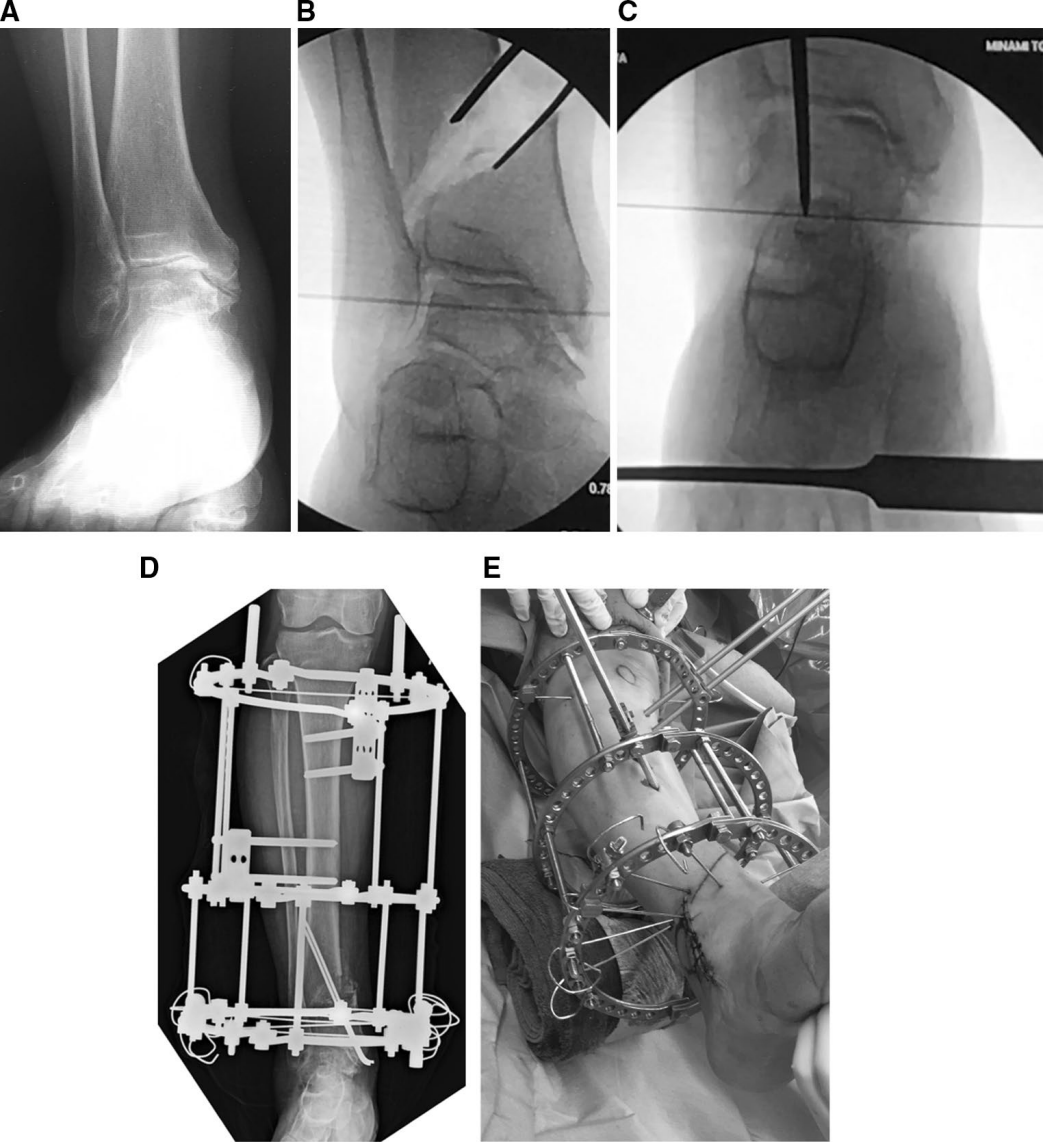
A 61-year-old woman with stage 3b of ankle osteoarthritis in her right ankle was treated with a DTOO. The weight-bearing radiograph before DTOO showed the joint space narrowing and the obliteration extended to the roof of the dome of the talus (a). The second radiograph shows separation of the osteotomy by laminar spreader until the lateral talar articular surface comes into contact with the distal medial fibular articular surface (b). Bony spurs present in the fibula and tibia are removed because of impingement during the corrective osteotomy. The final radiograph shows that the plantar surface of the foot becomes perpendicular to the tibial axis after osteotomy (shown by the overlying osteotome to indicate the tibial axis) (c). Fixation is with the Ilizarov external fixator (d, e)
